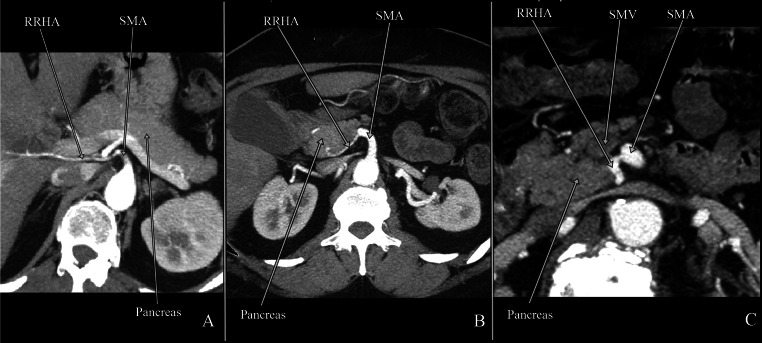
Computed tomography presenting three types of the RRHA. a
